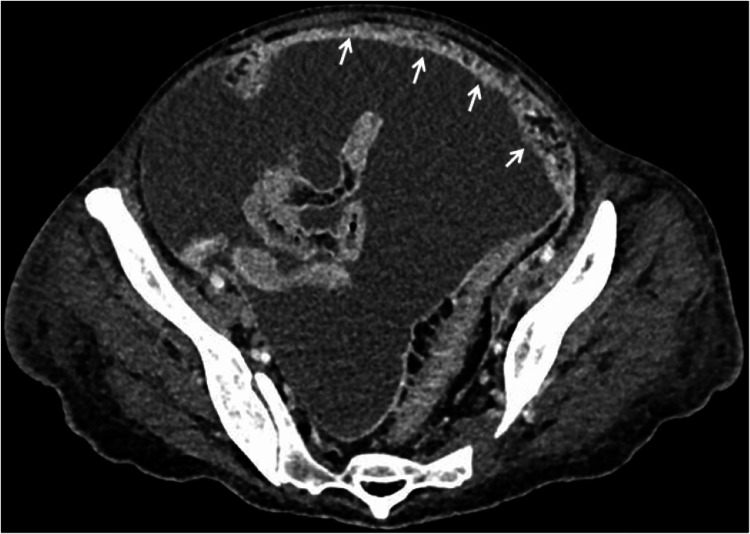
Abdominal and pelvic CT revealing tense ascites compressing the colon and aspects suggesting peritoneal carcinomatosis with an anterior epiploic “omental-cake” pattern (arrows)
